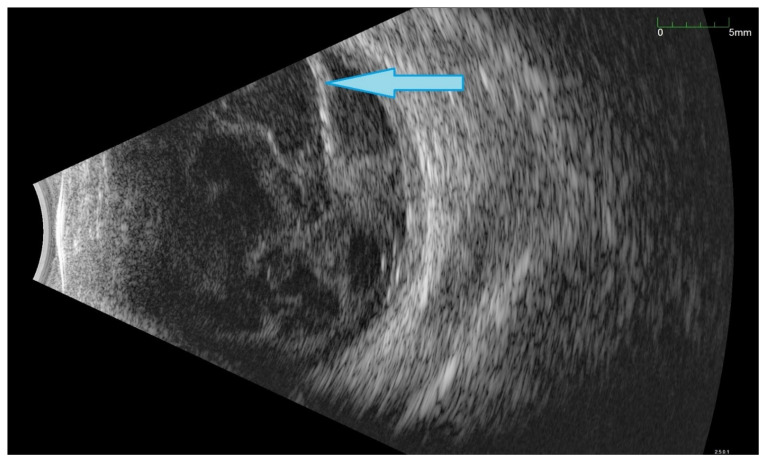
Ultrasound B scan with numerous hyperechoic densities in vitreous chamber. The blue arrow marks a detached retina. Hyperechoic masses are visible under the retina.
